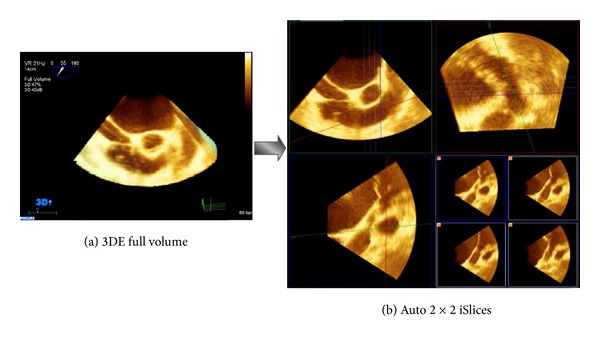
3D echocardiography dataset preparation including 3DE full volume acquisition (a) and 4-slices encompassing the RV of long-axis view using MPR mode in 3D QLAB software (b).
